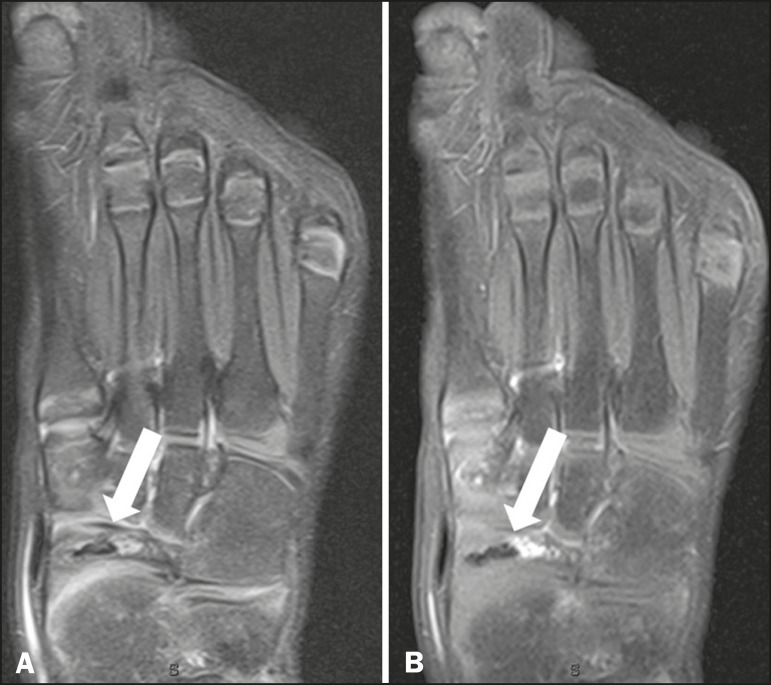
MRI of the same patient shown in Figure 6. A:
